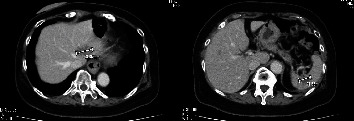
CT after 4 courses of TC therapy showed partial response. The size of the splenic tumor remained, but an increased size of the liver metastatic tumor was observed.
